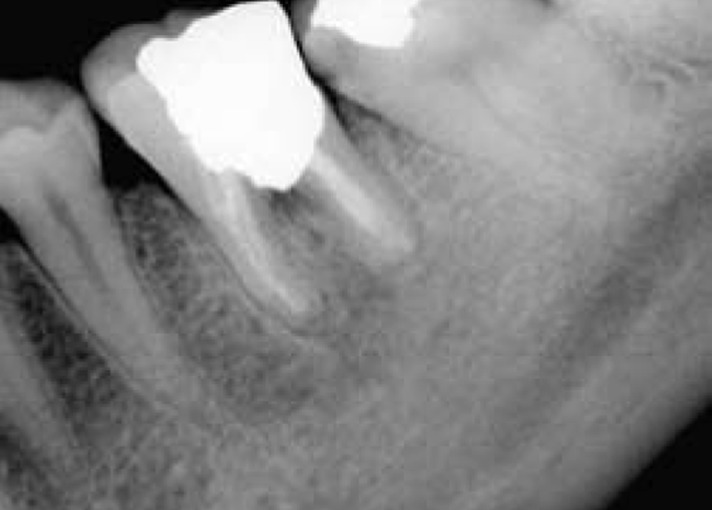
Two-year postoperative radiograph, PDL regeneration is evident.
